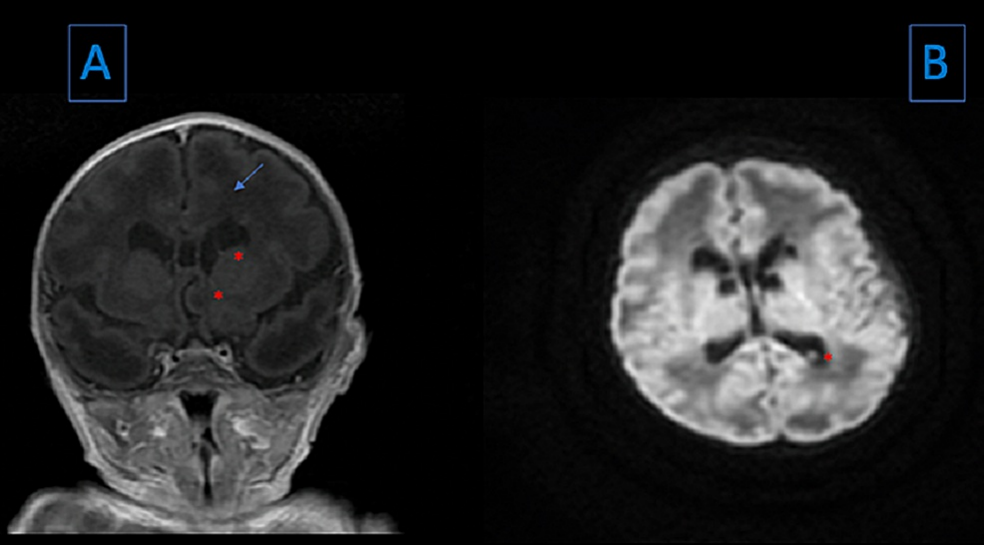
MRI findings Image A: MRI T1 sequence, coronal view. The blue line shows an enlargement of the lateral ventricles, the red asterisks show multiple periventricular/subependymal cysts in the caudothalamic grooves; Image B: MRI T2 FLAIR, sagittal view: the red asterisks show a subependymal cyst. MRI: magnetic resonance imaging; FLAIR: fluid-attenuated inversion recovery
